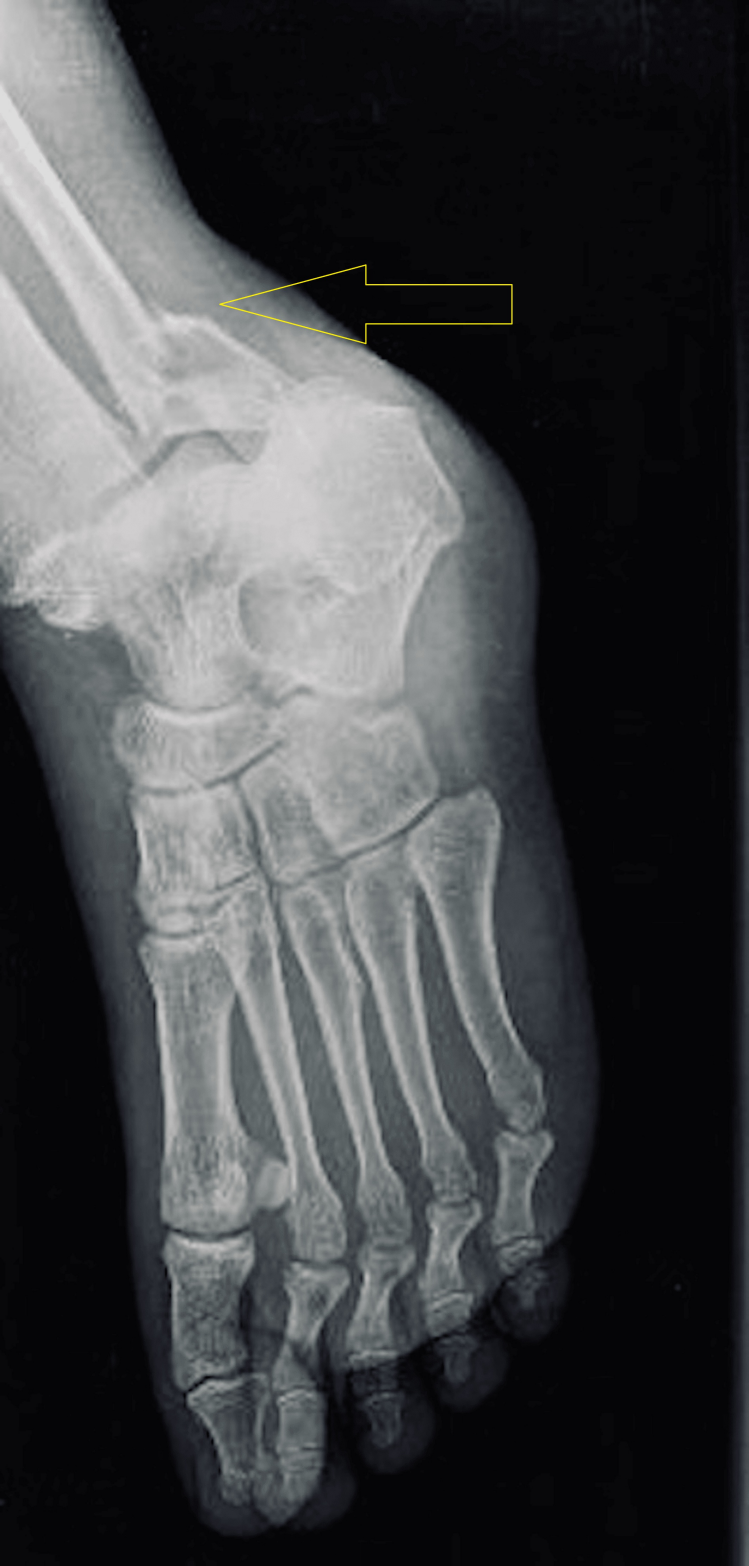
Pre-operative X-ray (mortise view) of the left ankle.
